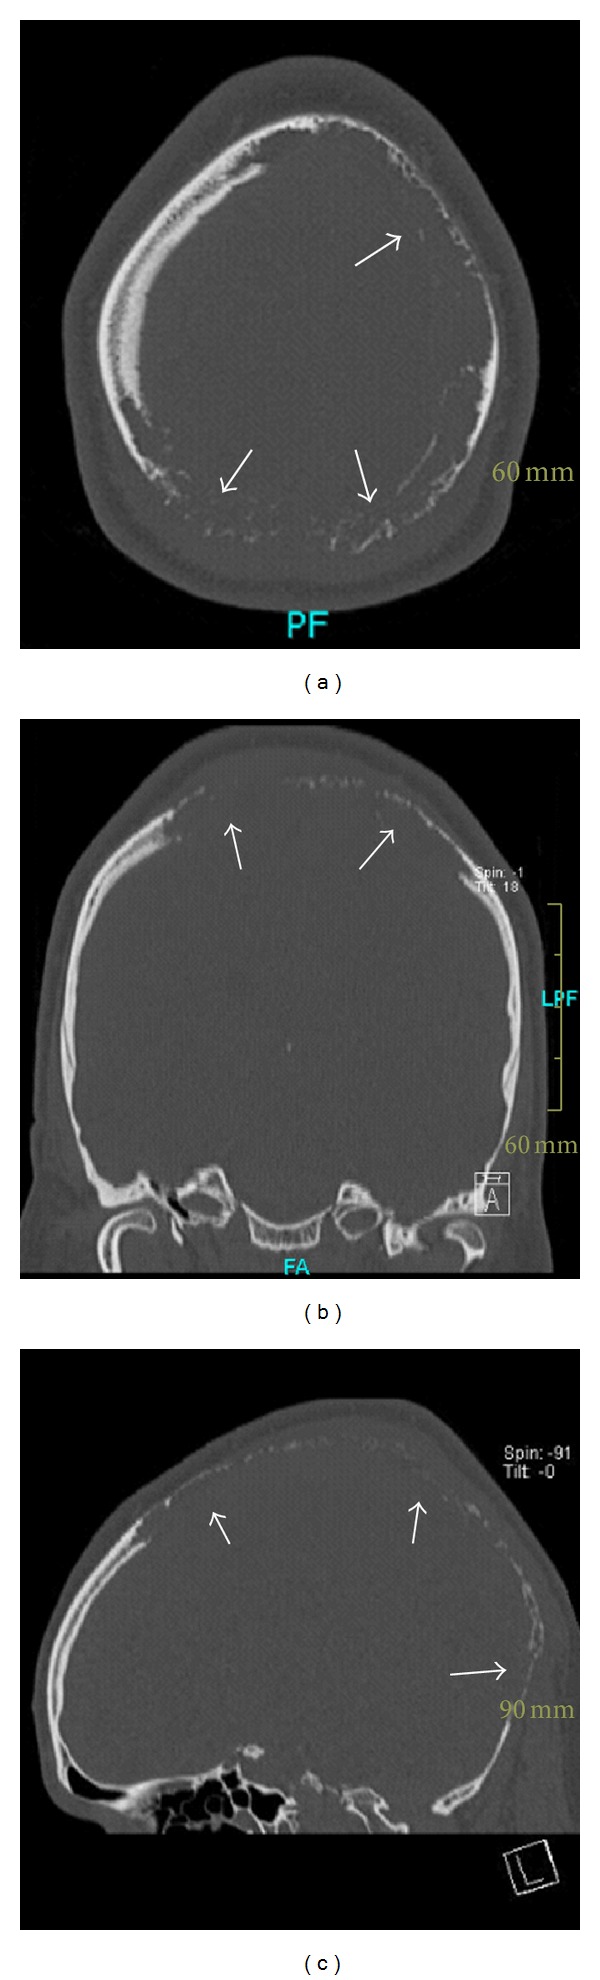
Cranial CT scan. (a) Axial view. (b, c) Coronar and sagittal reconstruction views. The cranial CT scan shows a massive bon destruction.
